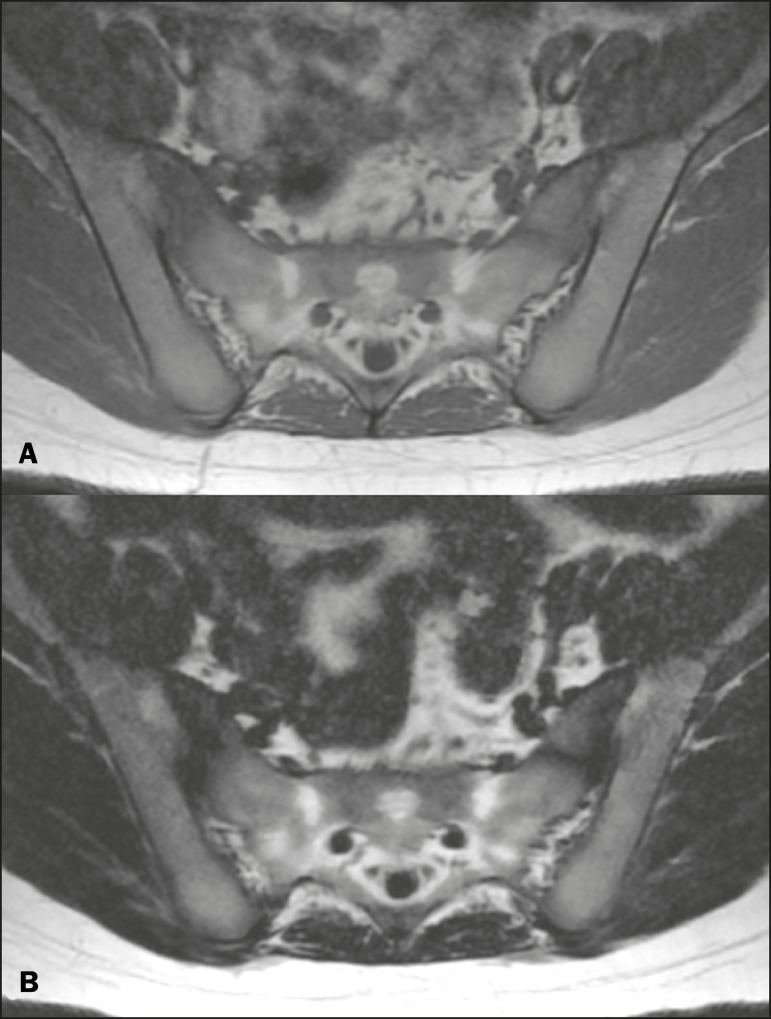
Axial images, from a T1-weighted FSE sequence (A) and a T2-weighted IDEAL fat-only sequence (B), of a 15-year-old female patient, showing periarticular areas of fatty replacement in the iliac components of both sacroiliac joints, detectable in both sequences.
